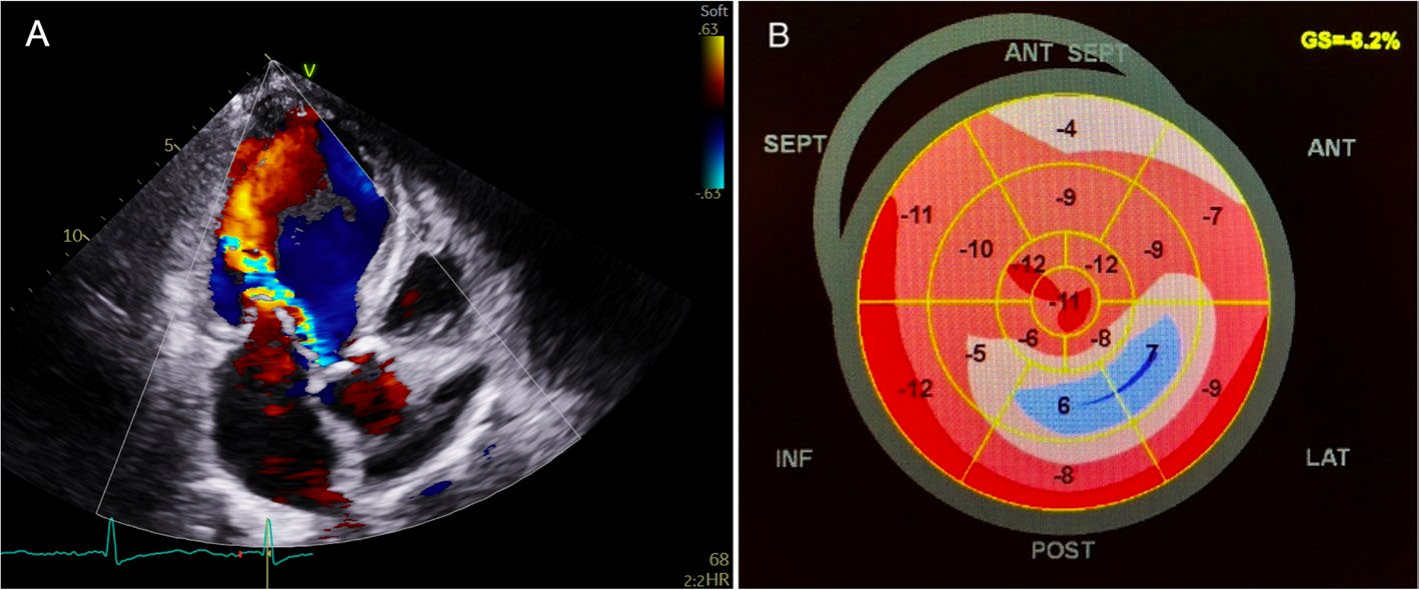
Example of a case of aortic regurgitation associated with jet-related strain reduction. (A) Apical three-chamber view showing an aortic regurgitant jet directed towards the mid inferolateral segment. (B) Longitudinal strain bull’s eye of the same patient showing worse longitudinal strain values in the mid inferolateral and anterolateral segments (impacted by the jet)
